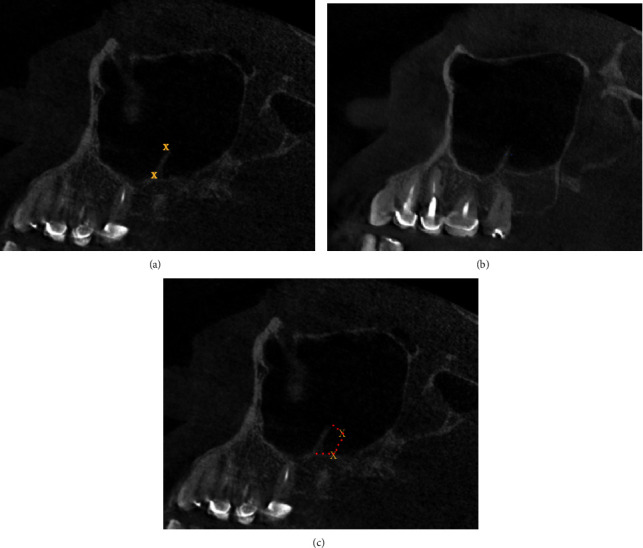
CBCT cuts showing the four points used in the calculation. Two medial points (blue) and two lateral points (blue).
